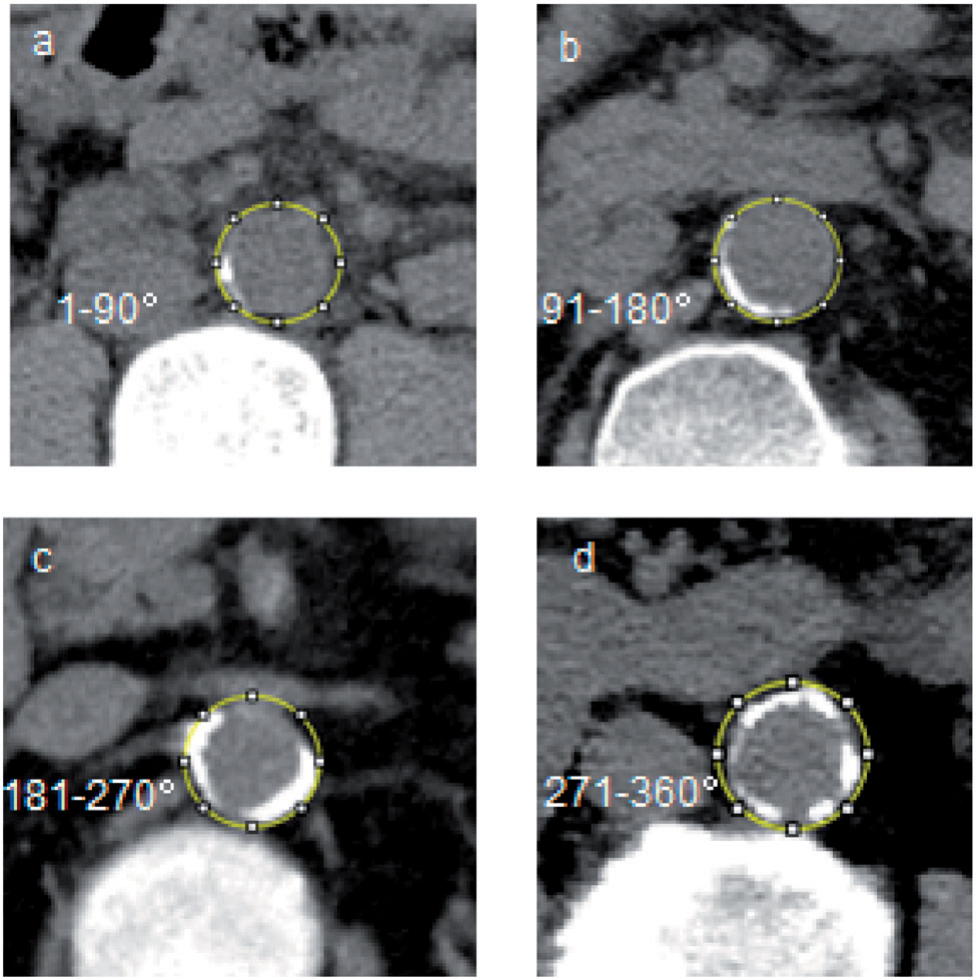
Cross-sectional view of abdominal aortic calcification by CT in four representative cases.
